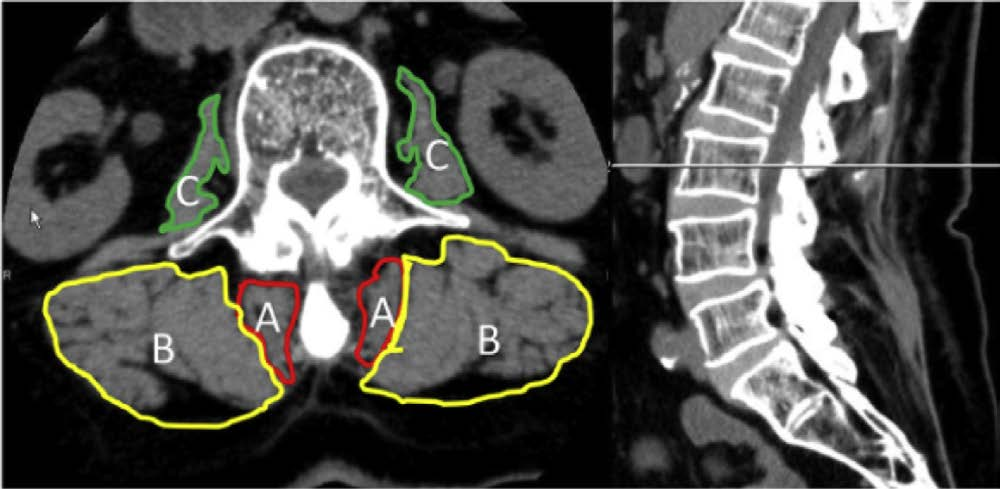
Kameyama et al^16]^ applied the summary method, as proposed by previous authors, to measure the cross-sectional area and muscle density on CT axial images of the paraspinal muscles. A: multifidus; B: erector spinae; C: lumbaris major. (Cited in Ref.^[16]^). CT = computed tomography.
